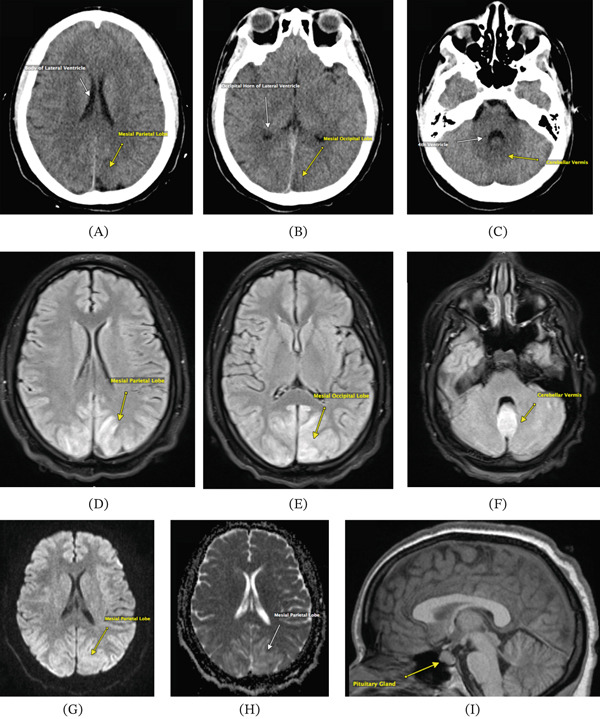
(A–C) Initial noncontrast axial CT of the head showed no abnormal cytotoxic or vasogenic edema. (D–F) Noncontrast axial MRI demonstrated symmetrical T2/FLAIR hyperintensities in the mesial parieto‐occipital lobes and cerebellar vermis bilaterally, consistent with vasogenic edema. (G–H) Diffusion‐weighted sequences showed increased DWI and ADC signal consistent with facilitated diffusion. (I) Noncontrast sagittal 3D T1 MPRAGE demonstrated nonspecific prominence of the pituitary gland without mass effect on the optic chiasm or suprasellar cistern.
